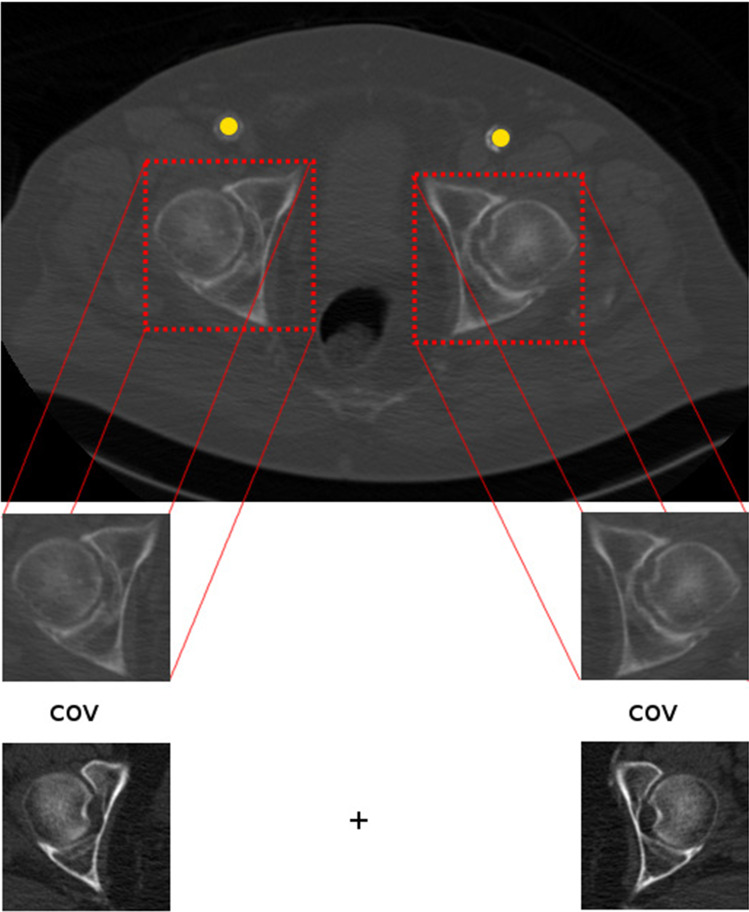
This figure visualizes how the template matching detects the caput femoris in the CT slice
